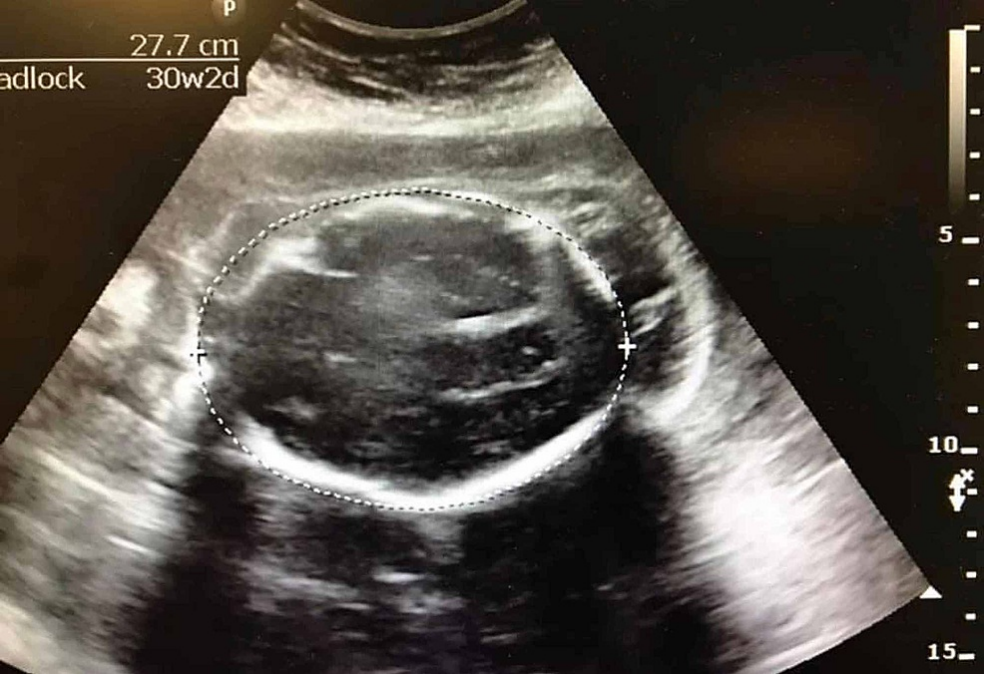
Fetal ultrasound, axial view. The dimension indicates the diameter of the head at 27.7 cm. Posterior aspect of the skull is depicted on the right side of the image.
